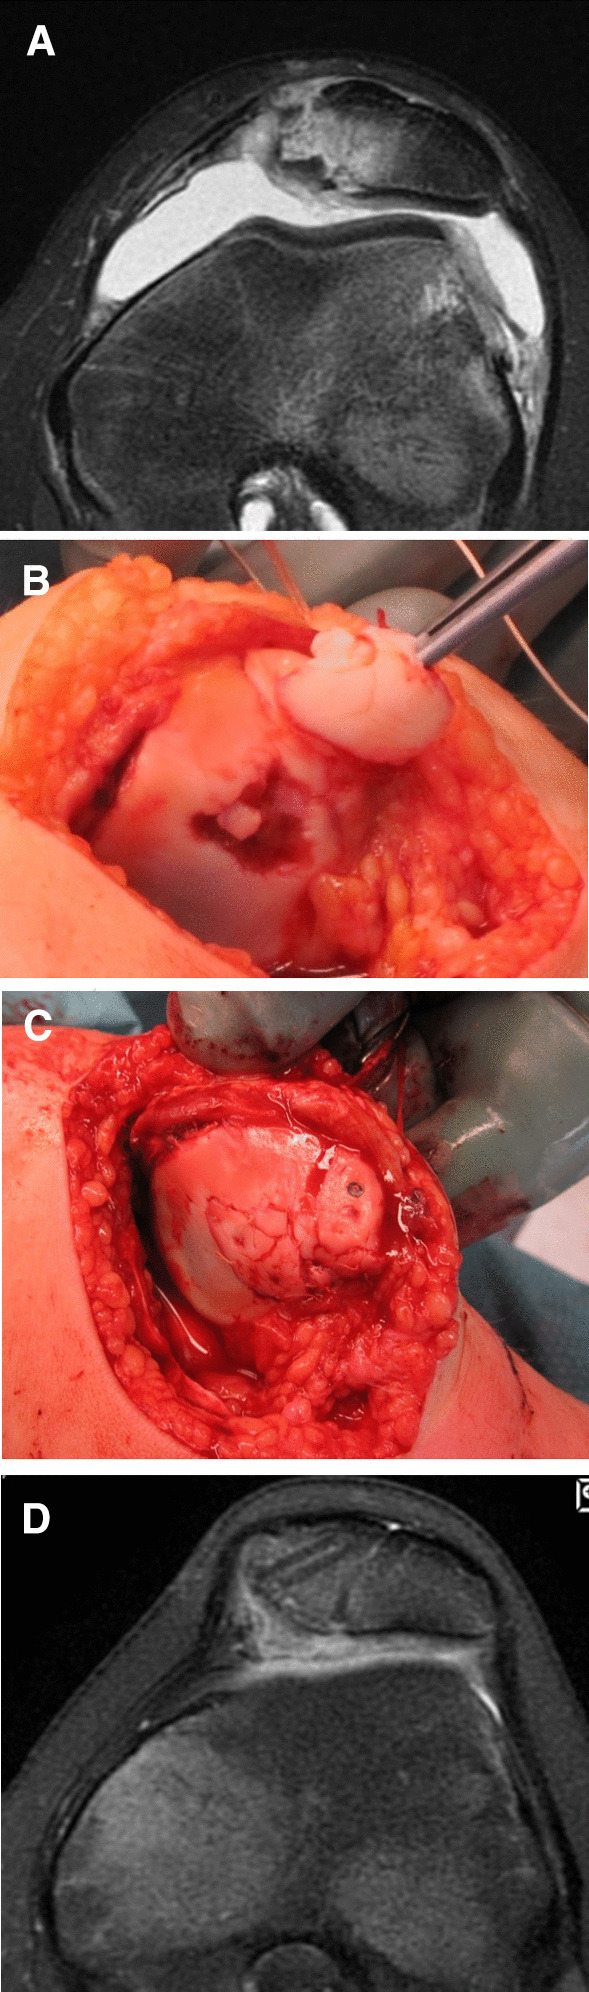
A Preoperative MRI of an osteochondral fracture on the medial facet of the patella in a 12-year-old girl who had sustained a patellar dislocation. B An intraoperative photograph of two large osteochondral fractures in the same patient. C Fixation of osteochondral fractures using rods and bioabsorbable sutures. An MPFL reconstruction was subsequently performed. D. Transverse MRI at 1 year follow-up of the same patient as in Fig. 3A–C aged 13 years showing the attached osteochondral fragments in place and the two tunnels where the ligament graft had been located. She had a mild J-sign, but a negative apprehension sign. There was no swelling of the knee, but some crepitus in forced extension. The knee was painless with normal ROM. This girl was able to play tennis and had no difficulties cycling
